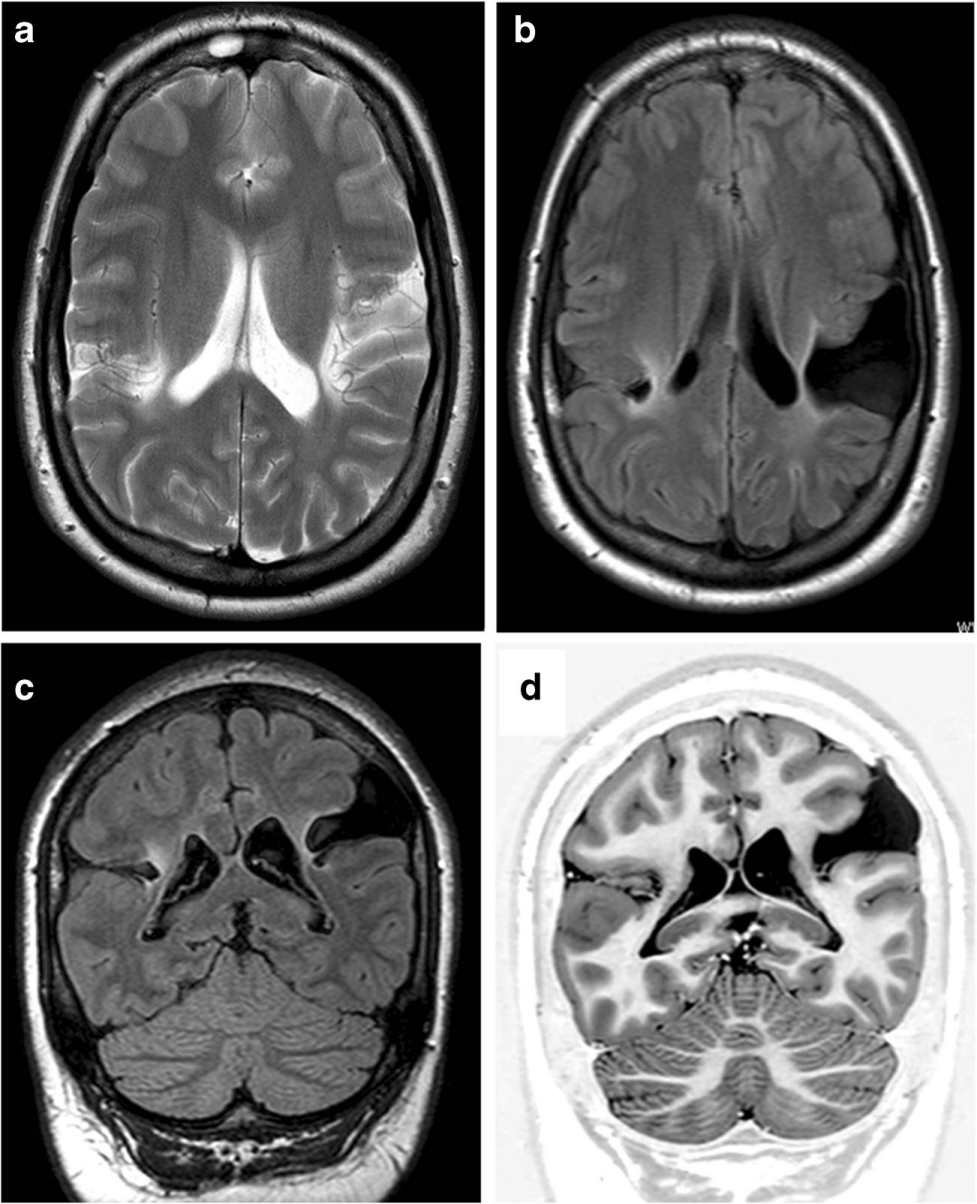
A child with porencephaly. MR imaging of an 8-year-old child with spastic quadriplegic cerebral palsy recognised in the first year of life. Axial T2-weighted (a), axial FLAIR (b), coronal FLAIR (c) and coronal inversion recovery (d) show bilateral clefts involving the paracentral lobules, which extend from the outer surface of the brain but do not quite reach the ventricular margin. Some of the white matter next to the clefts is gliotic and there is no evidence of normal, or abnormal, grey matter lining the clefts. These features indicate porencephaly rather than schizencephaly
